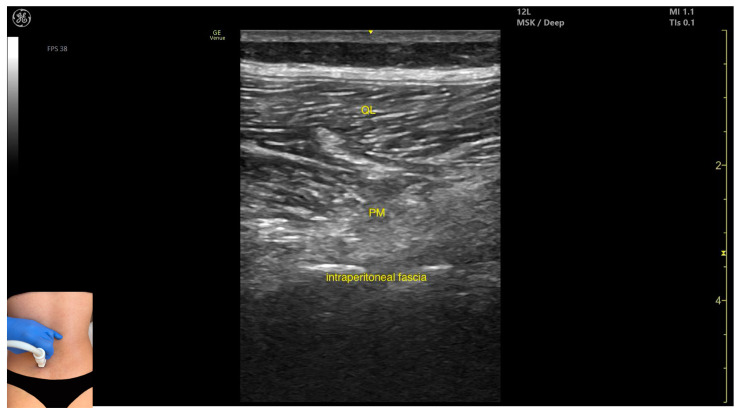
Ultrasound anatomy of *psoas major* (PM) (with the transducer longitudinally) with key landmarks: QL—*quadratus lumborum*; PM—psoas major.
